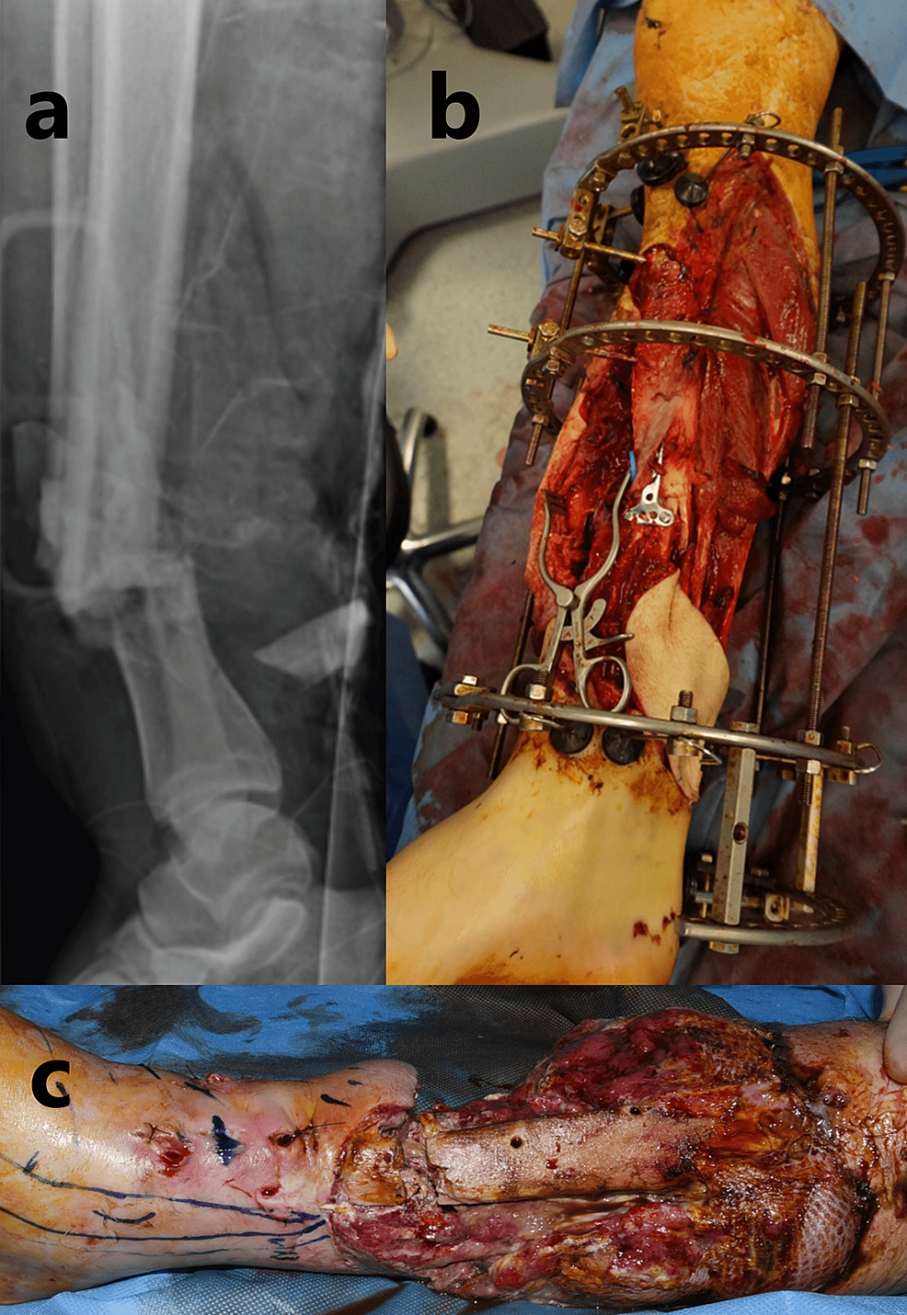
Unsuccessful treatment of the open fracture with fix and flap resulting in non-union: (a) radiograph of the lower limb open fracture, (b) intraoperative clinical photograph at the time of definitive fixation showing skin loss and method of fixation with frame and internal fixation, (c) intraoperative clinical photograph showing atrophic non-union with a large fracture gap and flap failure
